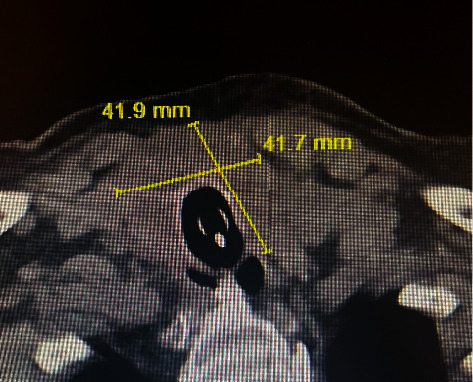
Following chemotherapy, the patient's CT neck scan with contrast showed a significant decrease in the thyroid size measured approximately 4.2 × 4.2 cm, with marked improvement in tracheal narrowing.
